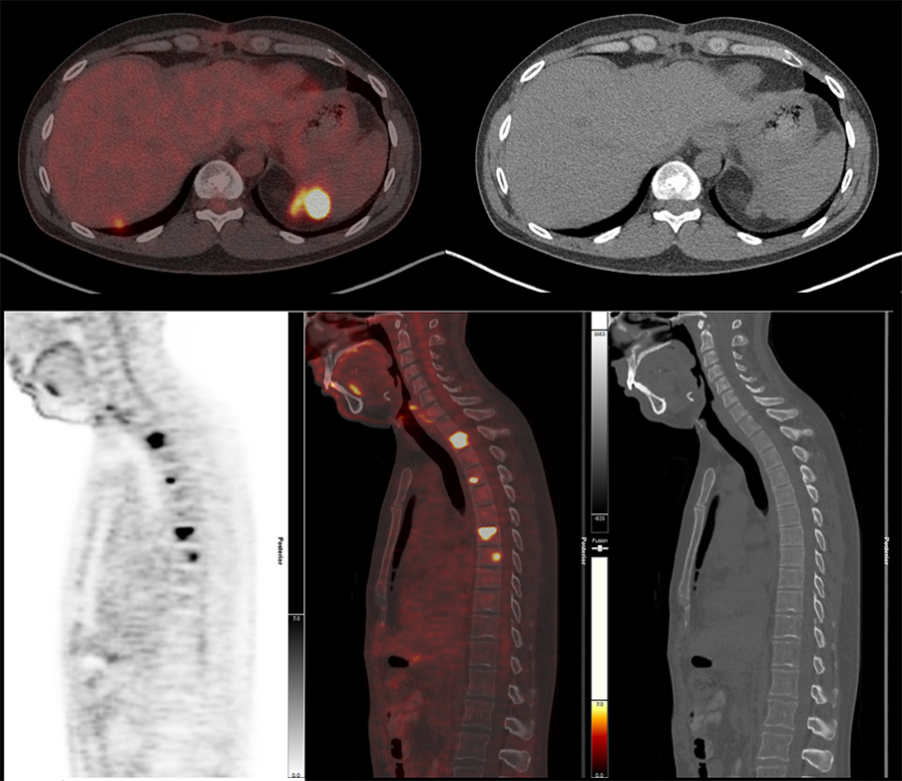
Post-surgery/chemotherapy positron emission tomography (bottom left), positron emission tomography/CT fusion (top left, bottom middle)/CT and CT images (top right, bottom right) showing new peritoneal and bone metastases.
